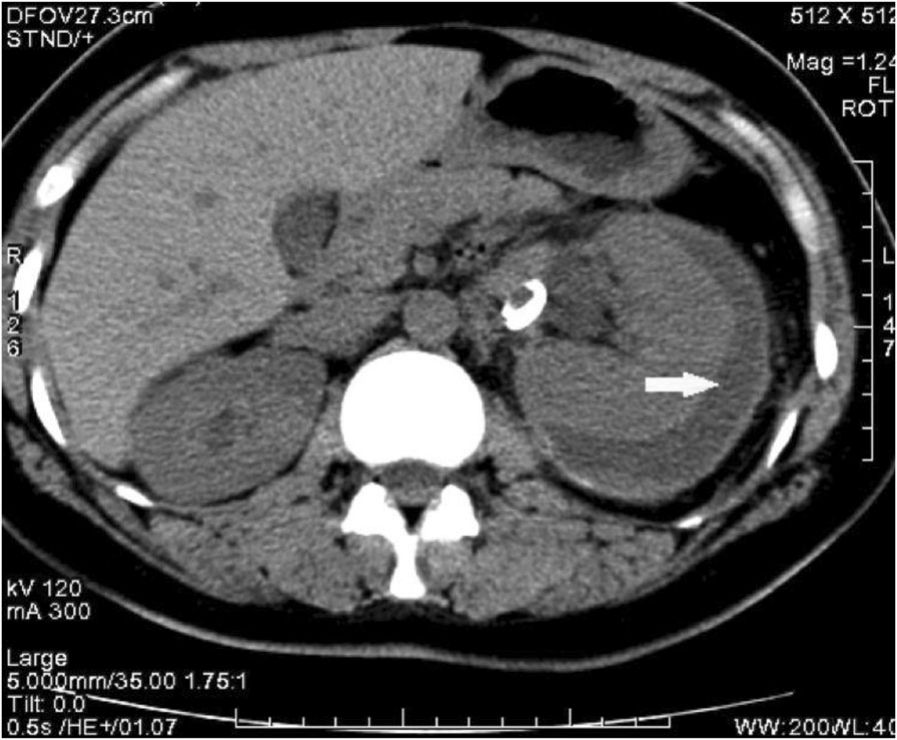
Two month after surgery the Coronal slice CT scan image showed the sub-capsular fluid (→)
